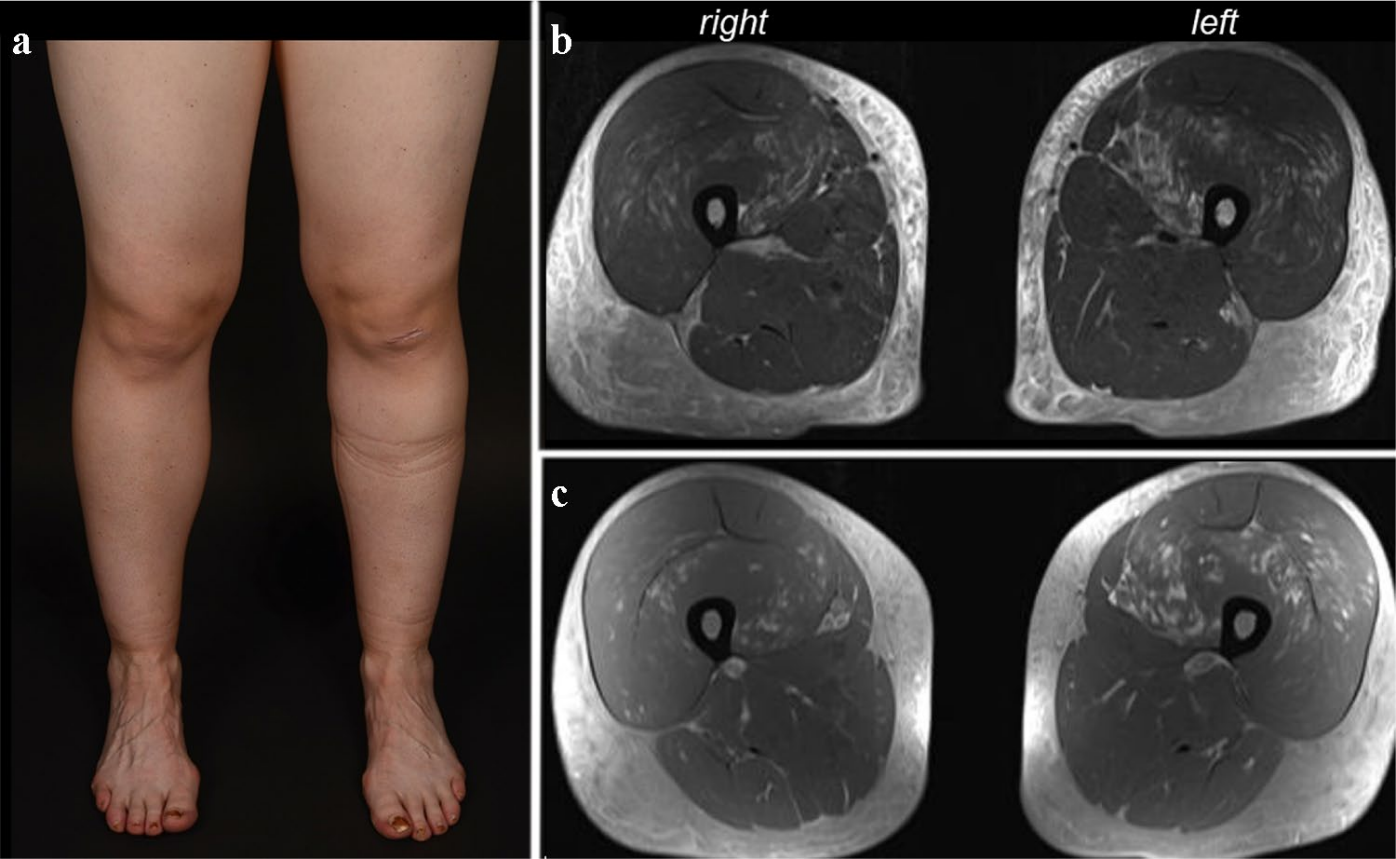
COVID-19 mRNA vaccine induced myopathy with rhabdomyolysis and fasciitis in a 28-year-old female. a Edematous swelling of both legs. b Transversal MRI of the thigh muscles in T2 and c T1 post-contrast shows left dominant signal alterations of the quadriceps muscles with contrast enhancement and subcutaneous edema suggestive of accompanying fasciitis
